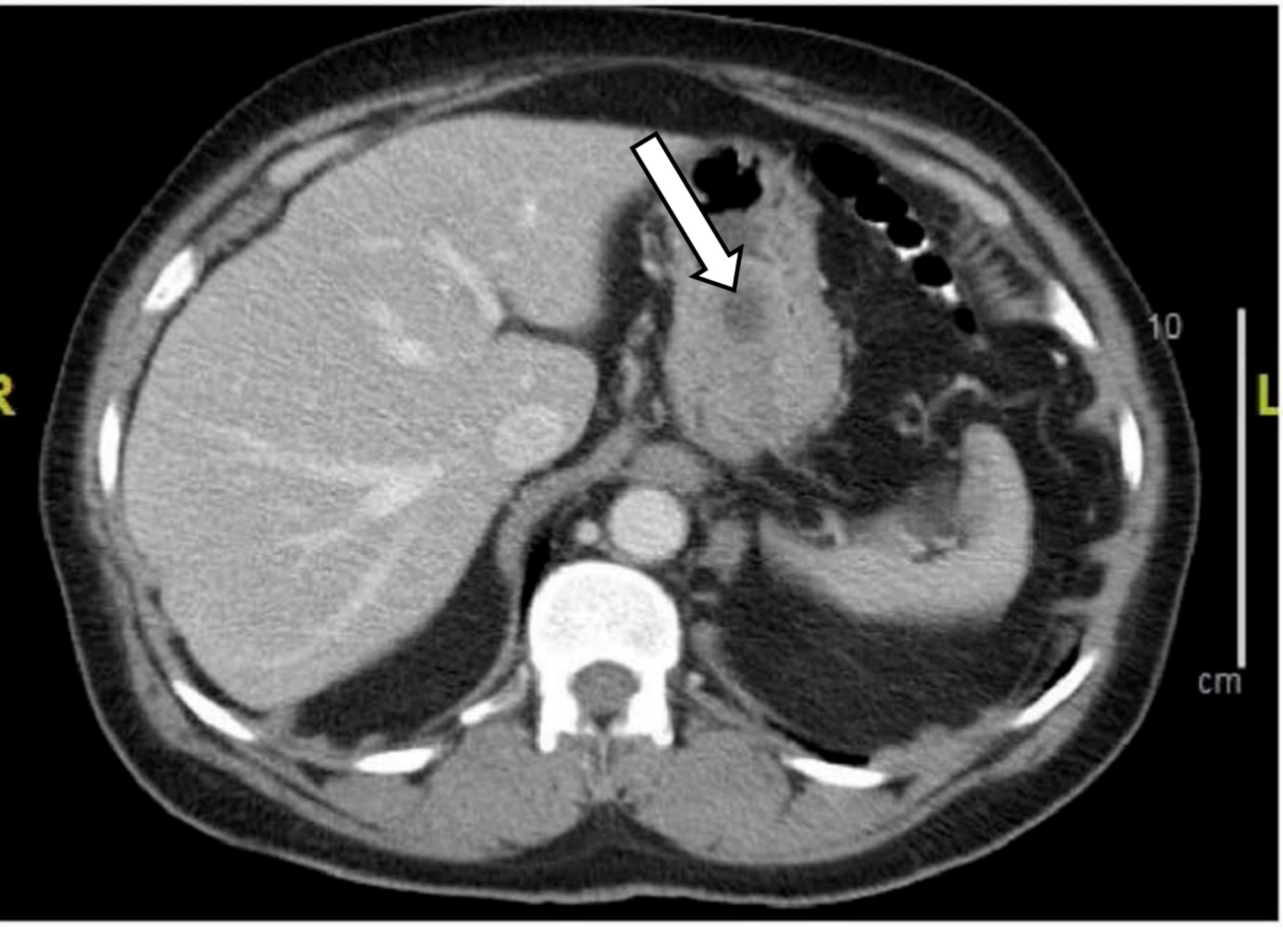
CT abdomen and pelvis with contrast material
